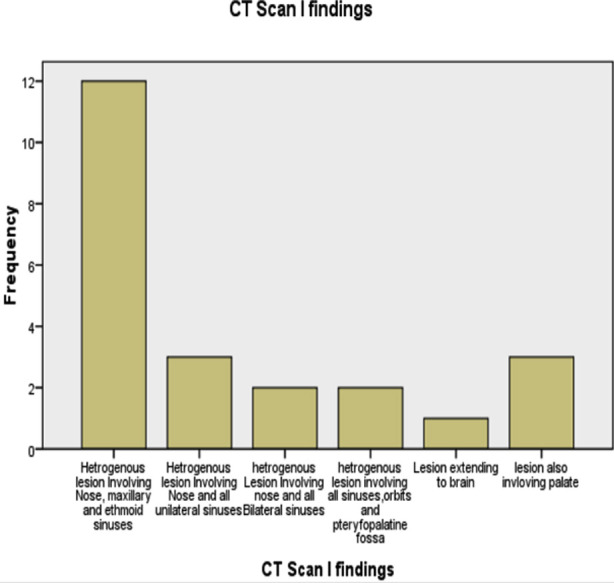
CT scan findings of patients with mucormycosis (n-23).
